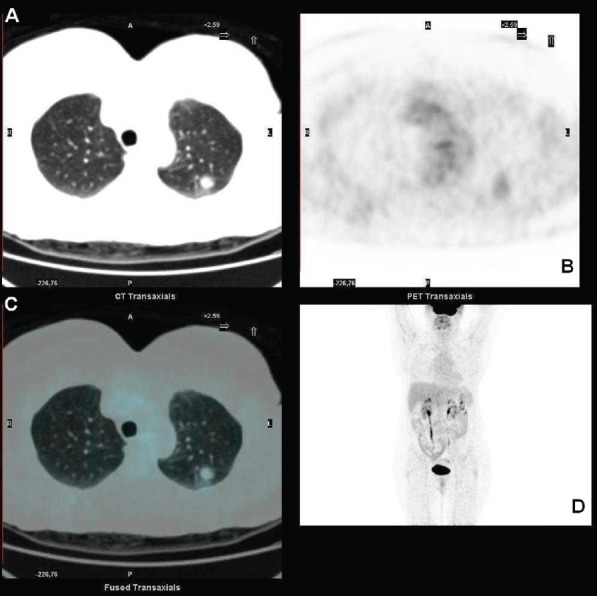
FDG-PET showing the pulmonary lesion in the upper lobe (a) transaxial low dose CT image, with midly increased metabolism (b) transaxial PET image, fused transaxial section and maximum intensity projection image (c and d).
